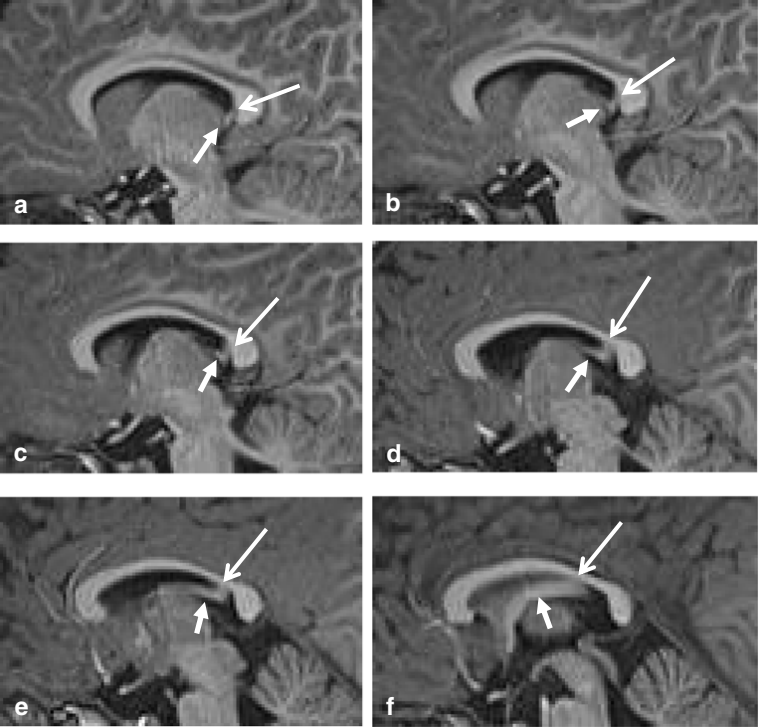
Sagittal 3D T1-weighted image (a–f) with short closed arrow pointing at the fornix and long open arrow indicating the hippocampal commissure, extending from the ventral splenium to the isthmus
